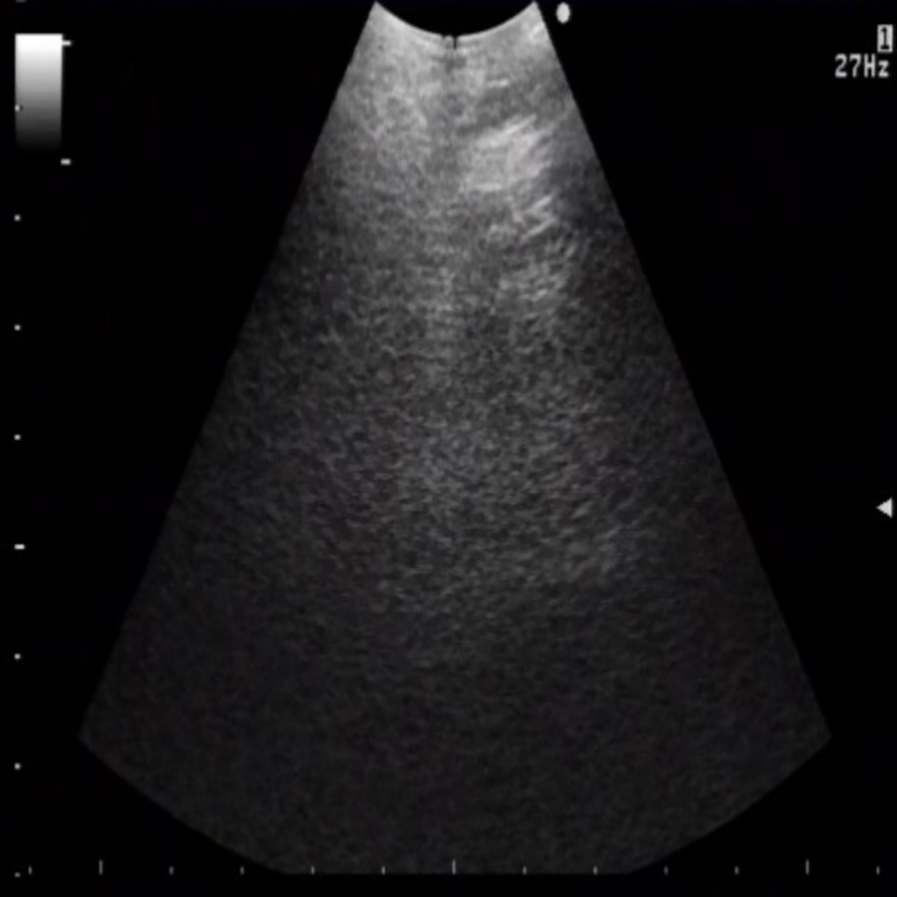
Axial ultrasound imaging: pre-hydrodissection.
